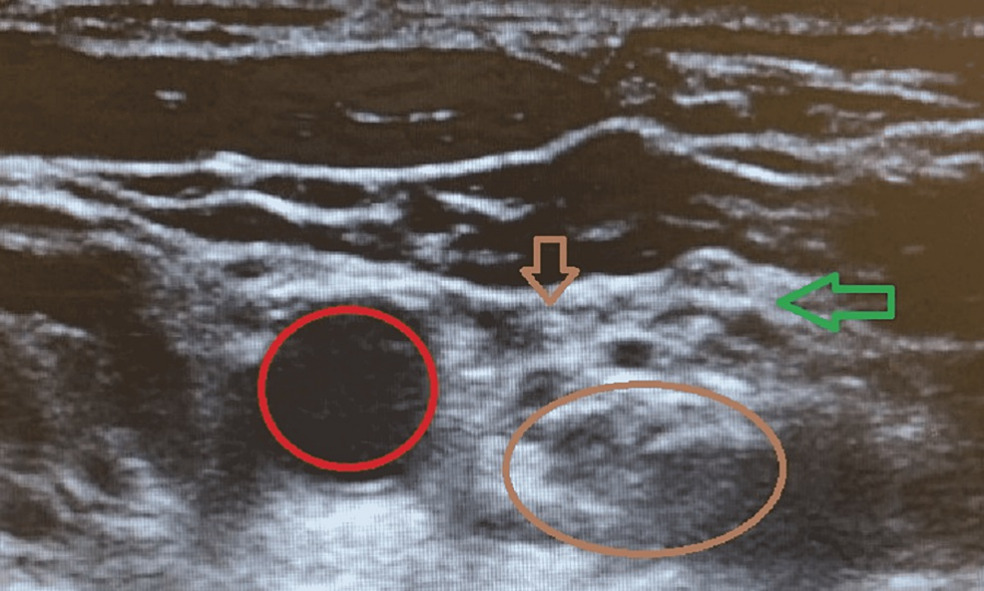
Ultrasound image of the brachial plexus nerve roots Red circle: carotid artery; brown circle: middle scalene muscle; green arrow: brachial plexus nerve roots; brown arrow: anterior scalene muscle
